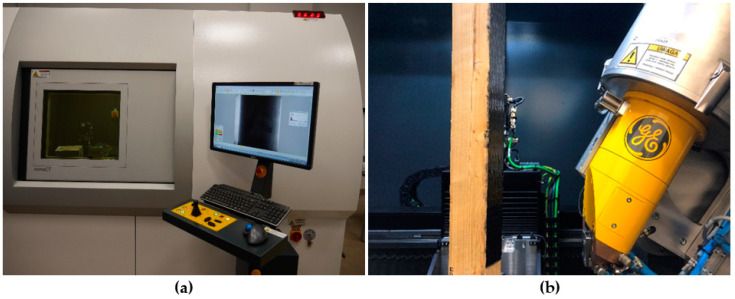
X-ray CT examinations: (a) the GE Phoenix v-tome-x m device during the test; (b) a sample prepared for tomographic examination mounted inside the device.
